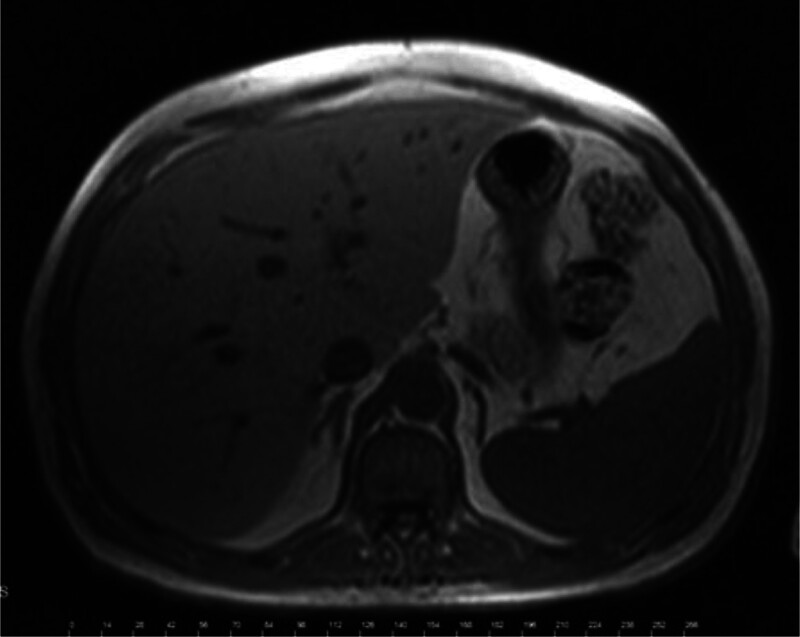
Abdominal MRI scan of the patient (hepatomegaly and hepatosteatosis were seen with normal adrenal glands and pancreas). MRI = magnetic resonance image.
